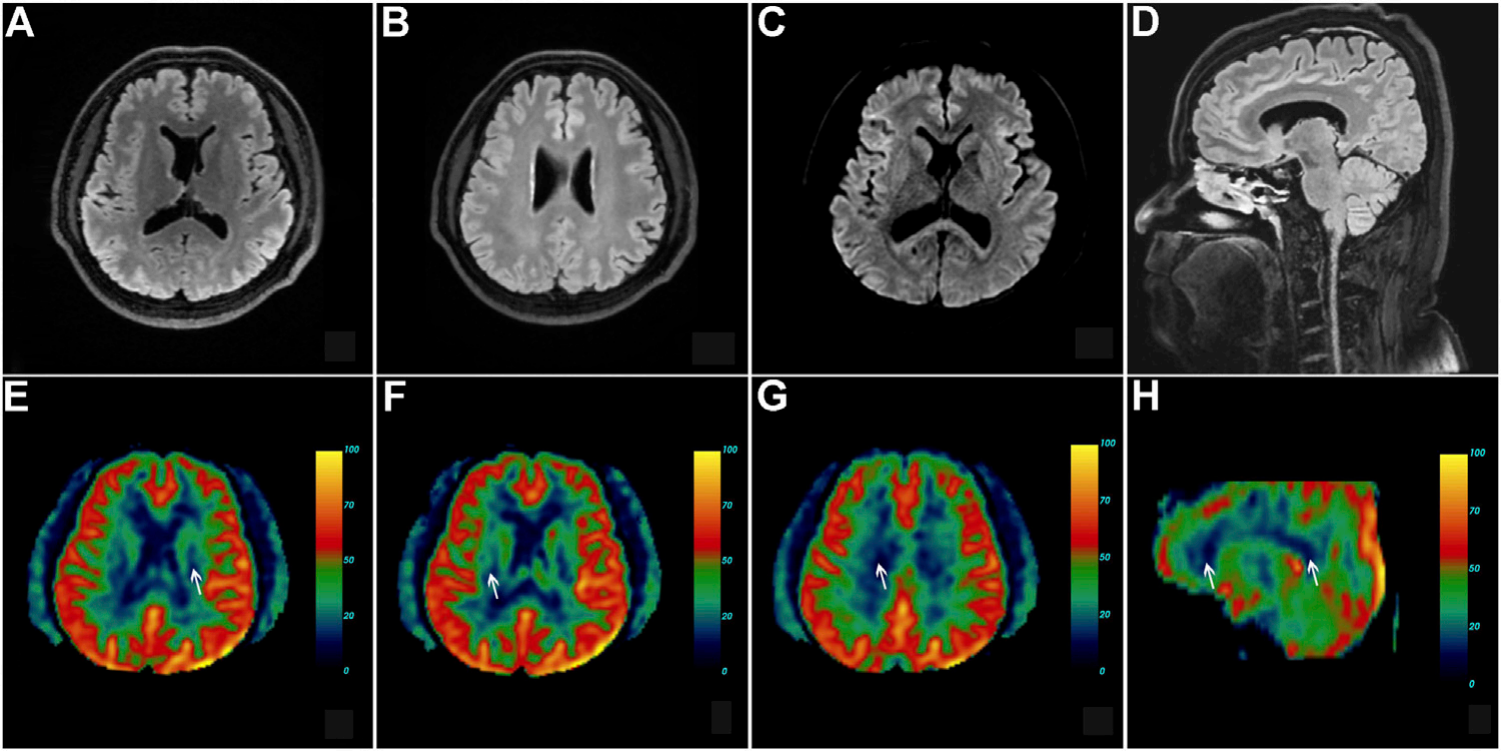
Brain neuroimaging of the proband’s brother (patient III-11) in the first family. Brain FLAIR demonstrates few slight hyperintensities in the periventricular white matter (A,B) and a focal high signal in the body of the corpus callosum (D). There is no abnormal signal in the axial diffusion-weighted imaging (C). Brain pseudo-continuous arterial spin labeling revealed a marked reduction of the cerebral blood flow (CBF) in the bilateral deep and subcortical white matter of frontoparietal lobes. The point value of the CBF in the left periventricular area is 15.3 ml/100 g/min (E), the point value of the CBF in the right periventricular area is 18.6 ml/100 g/min (F), the point value of the CBF in the subcortical deep white matter of parietal lobe is 8.6 ml/100 g/min (G), and the value of CBF in the anterior corpus callosum is 16.8 ml/100 g/min (H), while that in the splenium of corpus callosum is 13.5 ml/100 g/min.
